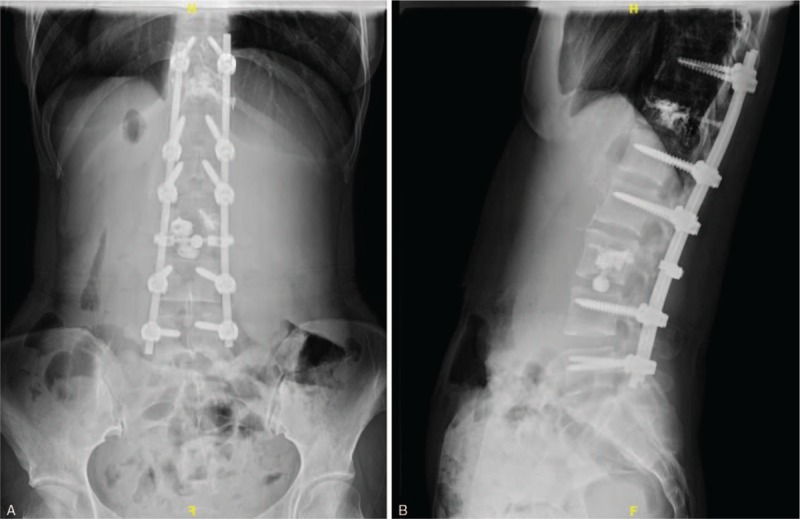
(A,B) Posteroanterior (PA) and lateral x-ray images of the lumbar spine obtained postoperatively.
